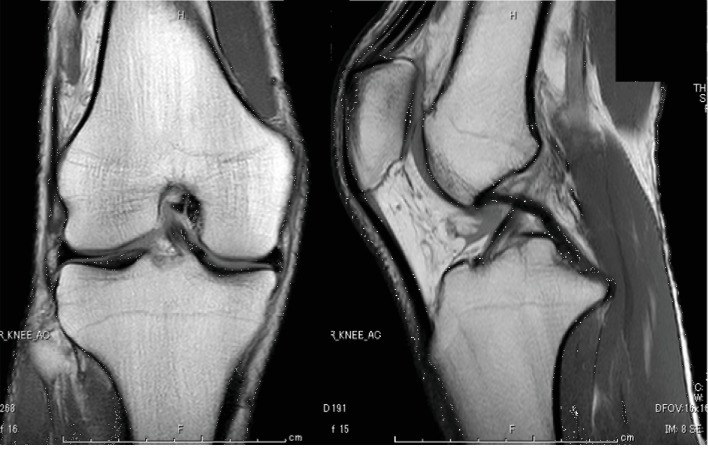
Magnetic resonance imaging of the knee. There was no evidence of intra- or extra-articular abnormalities.
